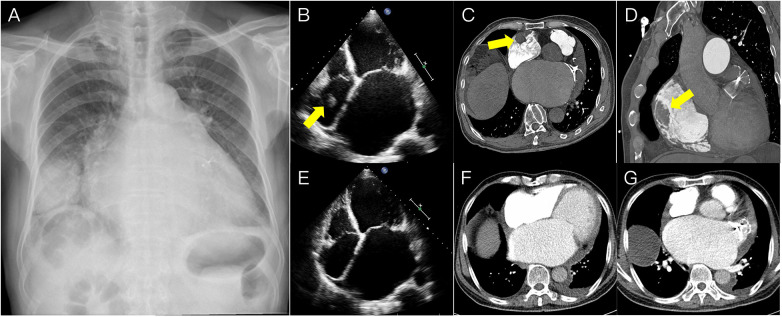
Imaging findings of right atrial appendage thrombosis and its resolution. (A) Chest radiograph demonstrating cardiomegaly with the left atrial appendage occluder device visible within the cardiac silhouette. (B) Transthoracic echocardiography showing a mobile mass (30 × 19 mm, arrow) in the right atrium. (C) and (D) Contrast-enhanced cardiac computed tomography confirming a filling defect within the right atrial appendage (arrows). (E) Transthoracic echocardiography at two weeks demonstrating complete resolution. (F) and (G) Contrast-enhanced cardiac CT at two weeks demonstrating resolution.
